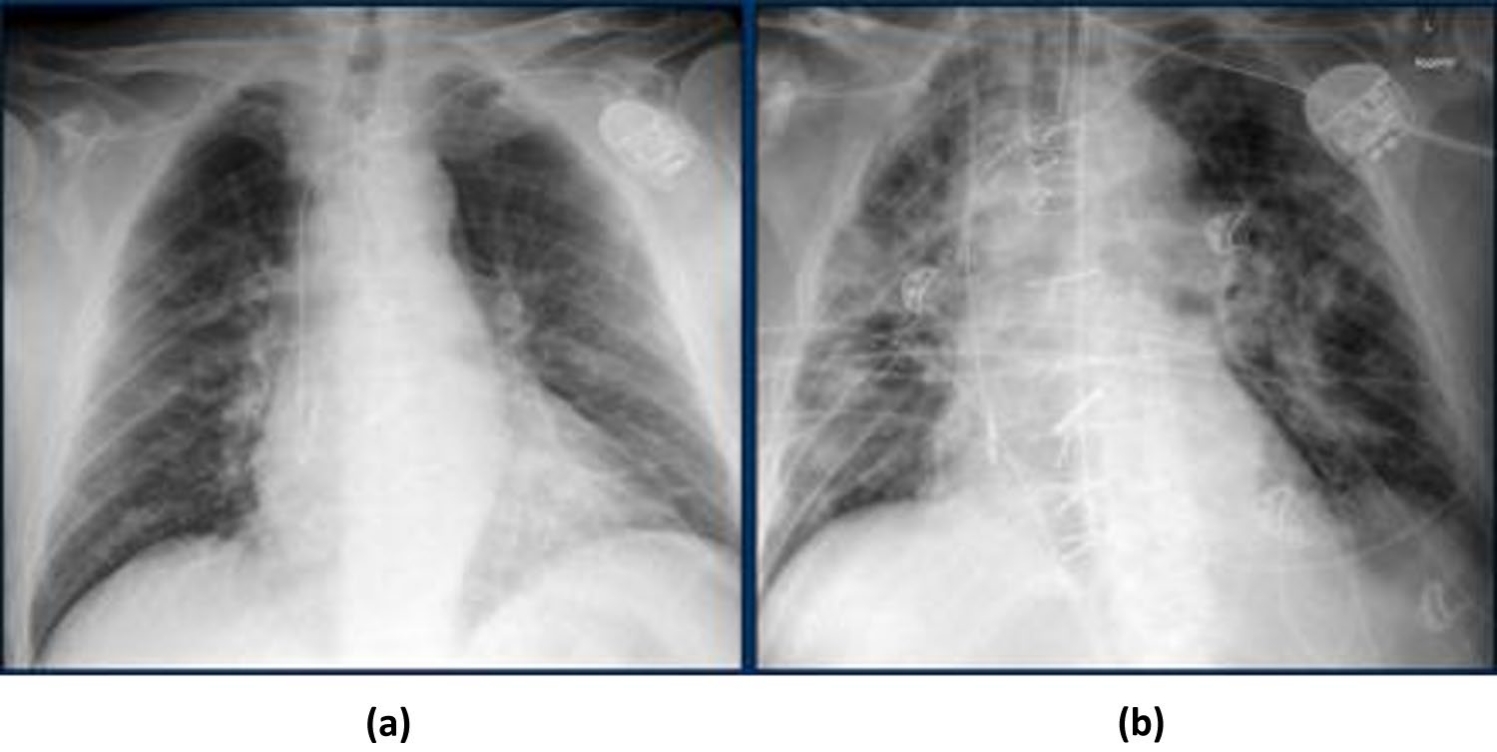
X-ray Images of COVID-19 Patient in (a) Non-critical and (b) Critical Condition
